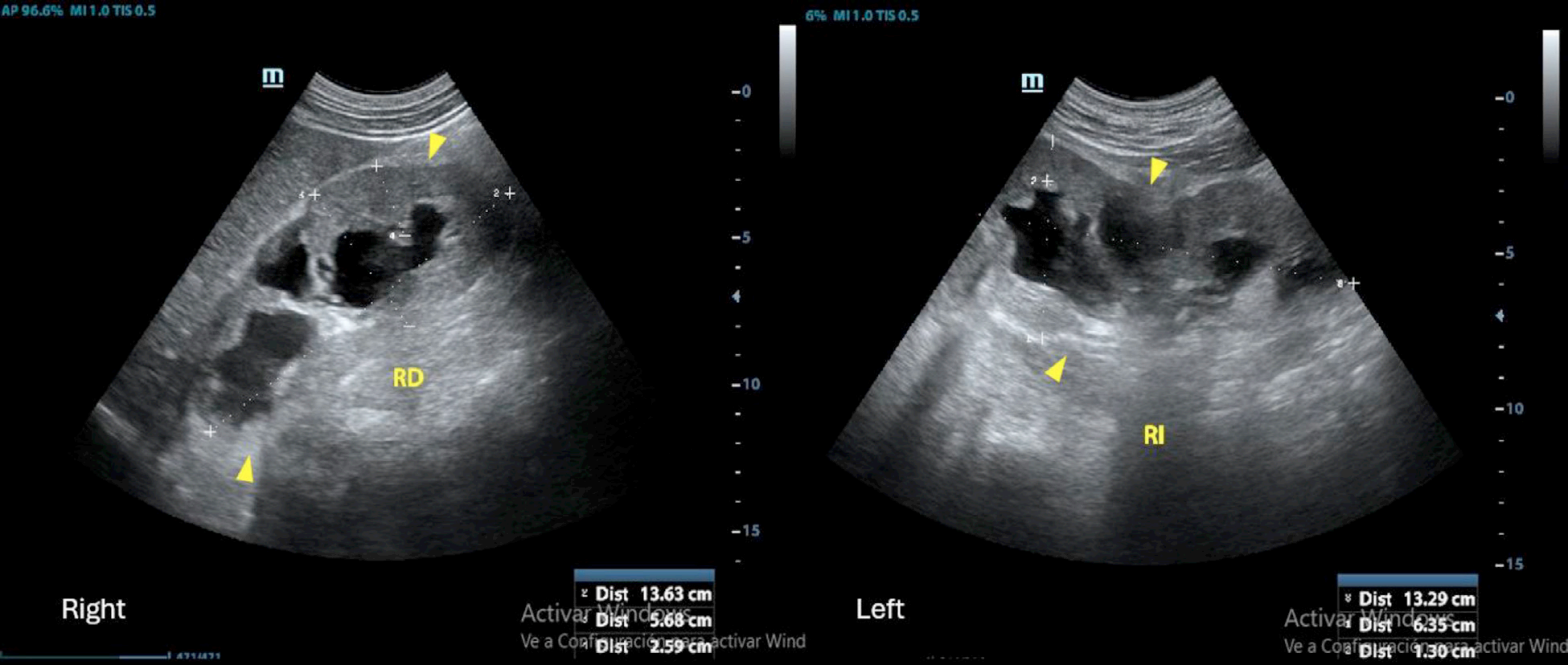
Postsurgical renal ultrasound. Mild and bilateral decrease in renal dimensions with signs of interstitial nephropathy .
